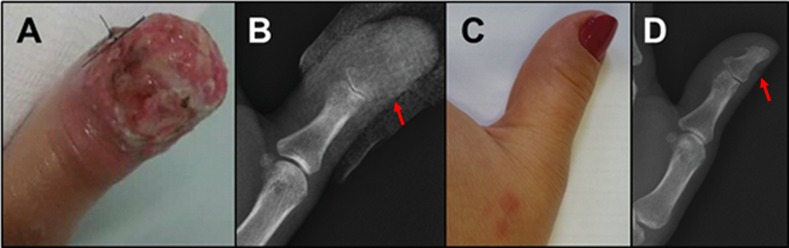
Unifocal form (case 12). A) Exuberant ulcerated lesion of fixed cutaneous sporotrichosis, with first right finger volume increase. B) Radiography—Destruction of the distal phalanx of the first right finger, with swelling of soft tissue (arrow). C-D) Clinical and radiological improvement after 9 months (arrow). (Source: A, C—Laboratory of Clinical Research in Infectious Dermatology; B, D—Service of Image of the INI).
